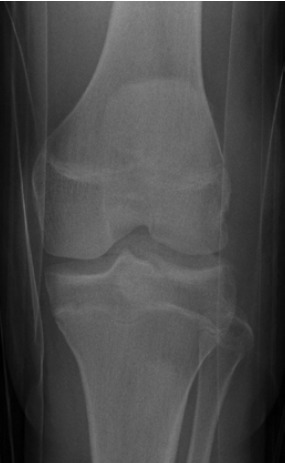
Preoperative anteroposterior radiograph of the left knee.
